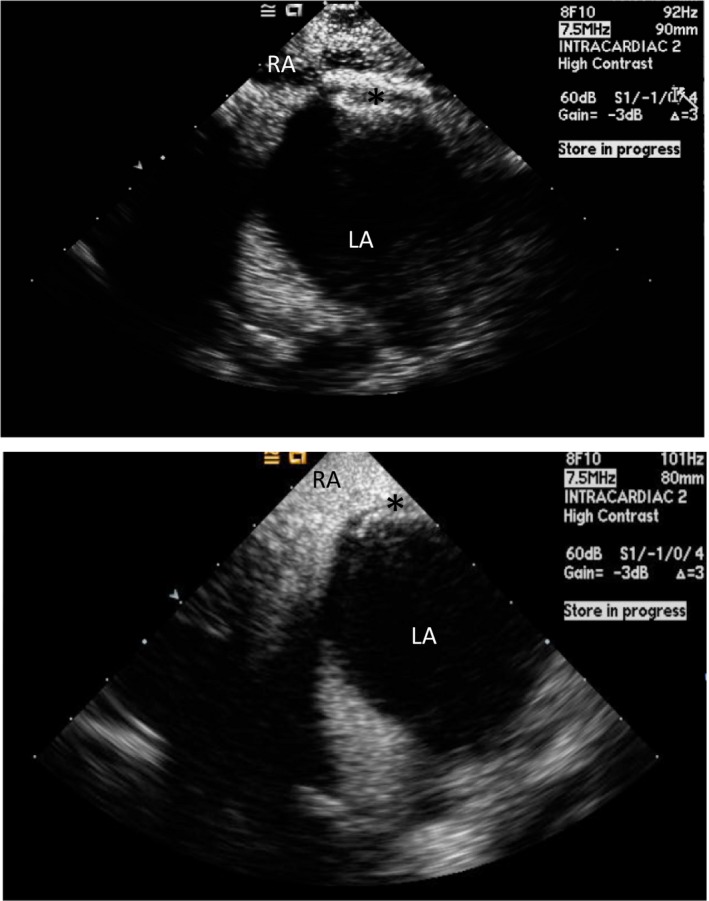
Intracardiac echocardiogram (ICE) image of a released Amplatzer PFO Occluder (top) (*) and Gore Cardioform Septal Occluder (bottom) (*). Agitated saline is seen in the right atrium (RA), but not in the left atrium (LA), confirming the absence of right to left shunt after device deployment.
